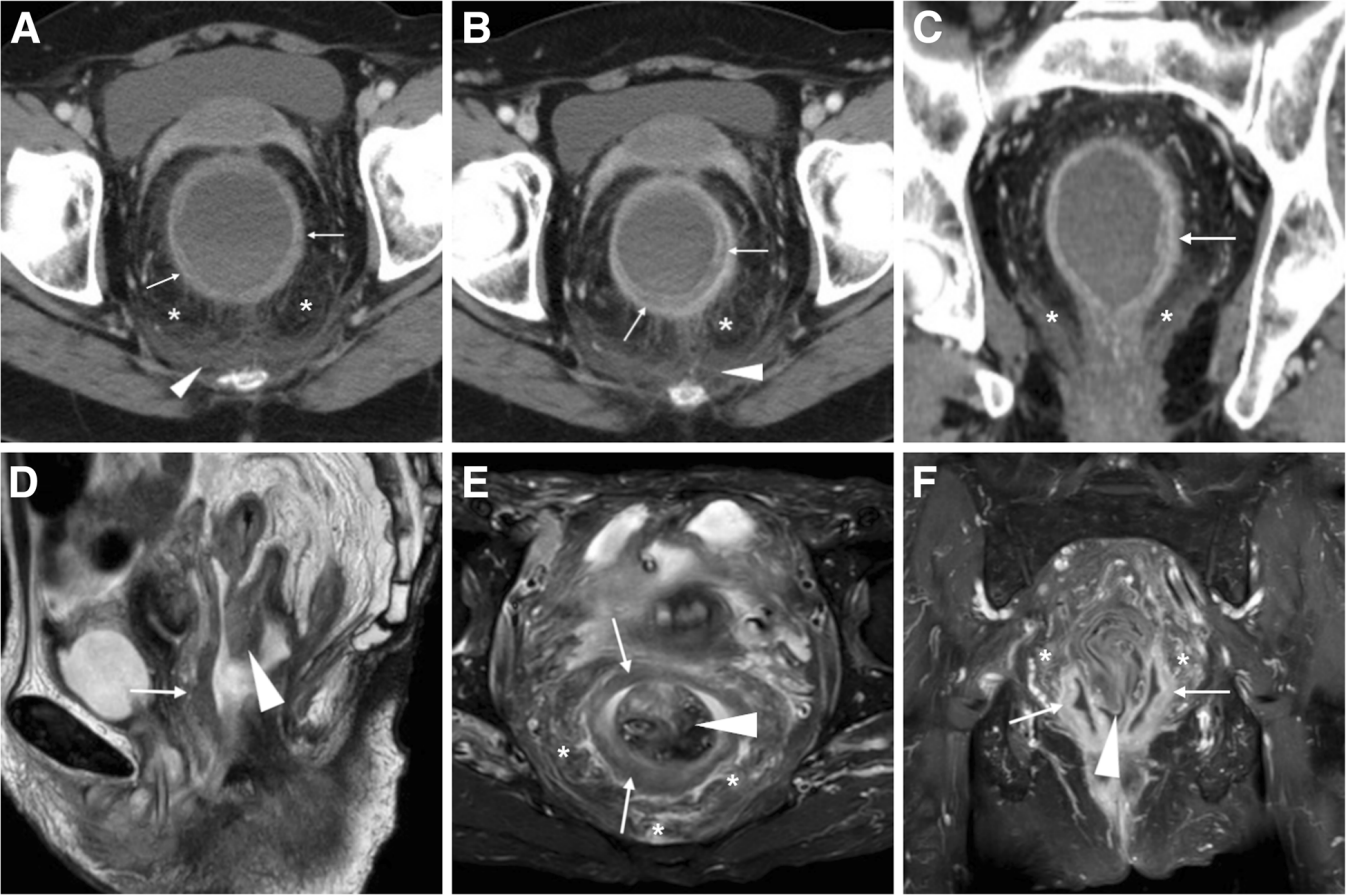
Two cases of diversion proctitis. a-c) a year after colectomy and terminal ileostomy for Crohn’s disease, initial CT (a) performed for perineal pain showed fluid-filled inflamed rectal stump with uniformly thick walls (thin arrows), mesorectal engorgement (*) and presacral fluid (arrowhead). Despite transanal drainage of fluid, repeated CT three months later (b-c) showed increased thickness and appearance of stratification at inflamed rectal walls (thin arrows). d-f) following colonic resection for indeterminate colitis, MRI including sagittal T2- (a), fat-suppressed axial T2- and post-gadolinium fat-suppressed T1-weighted (C) sequences showed markedly thickened oedematous and hypervascular rectal walls (thin arrows), perirectal fluid (*) and downwards invagination of the closed apex of rectal stump (arrowheads)
